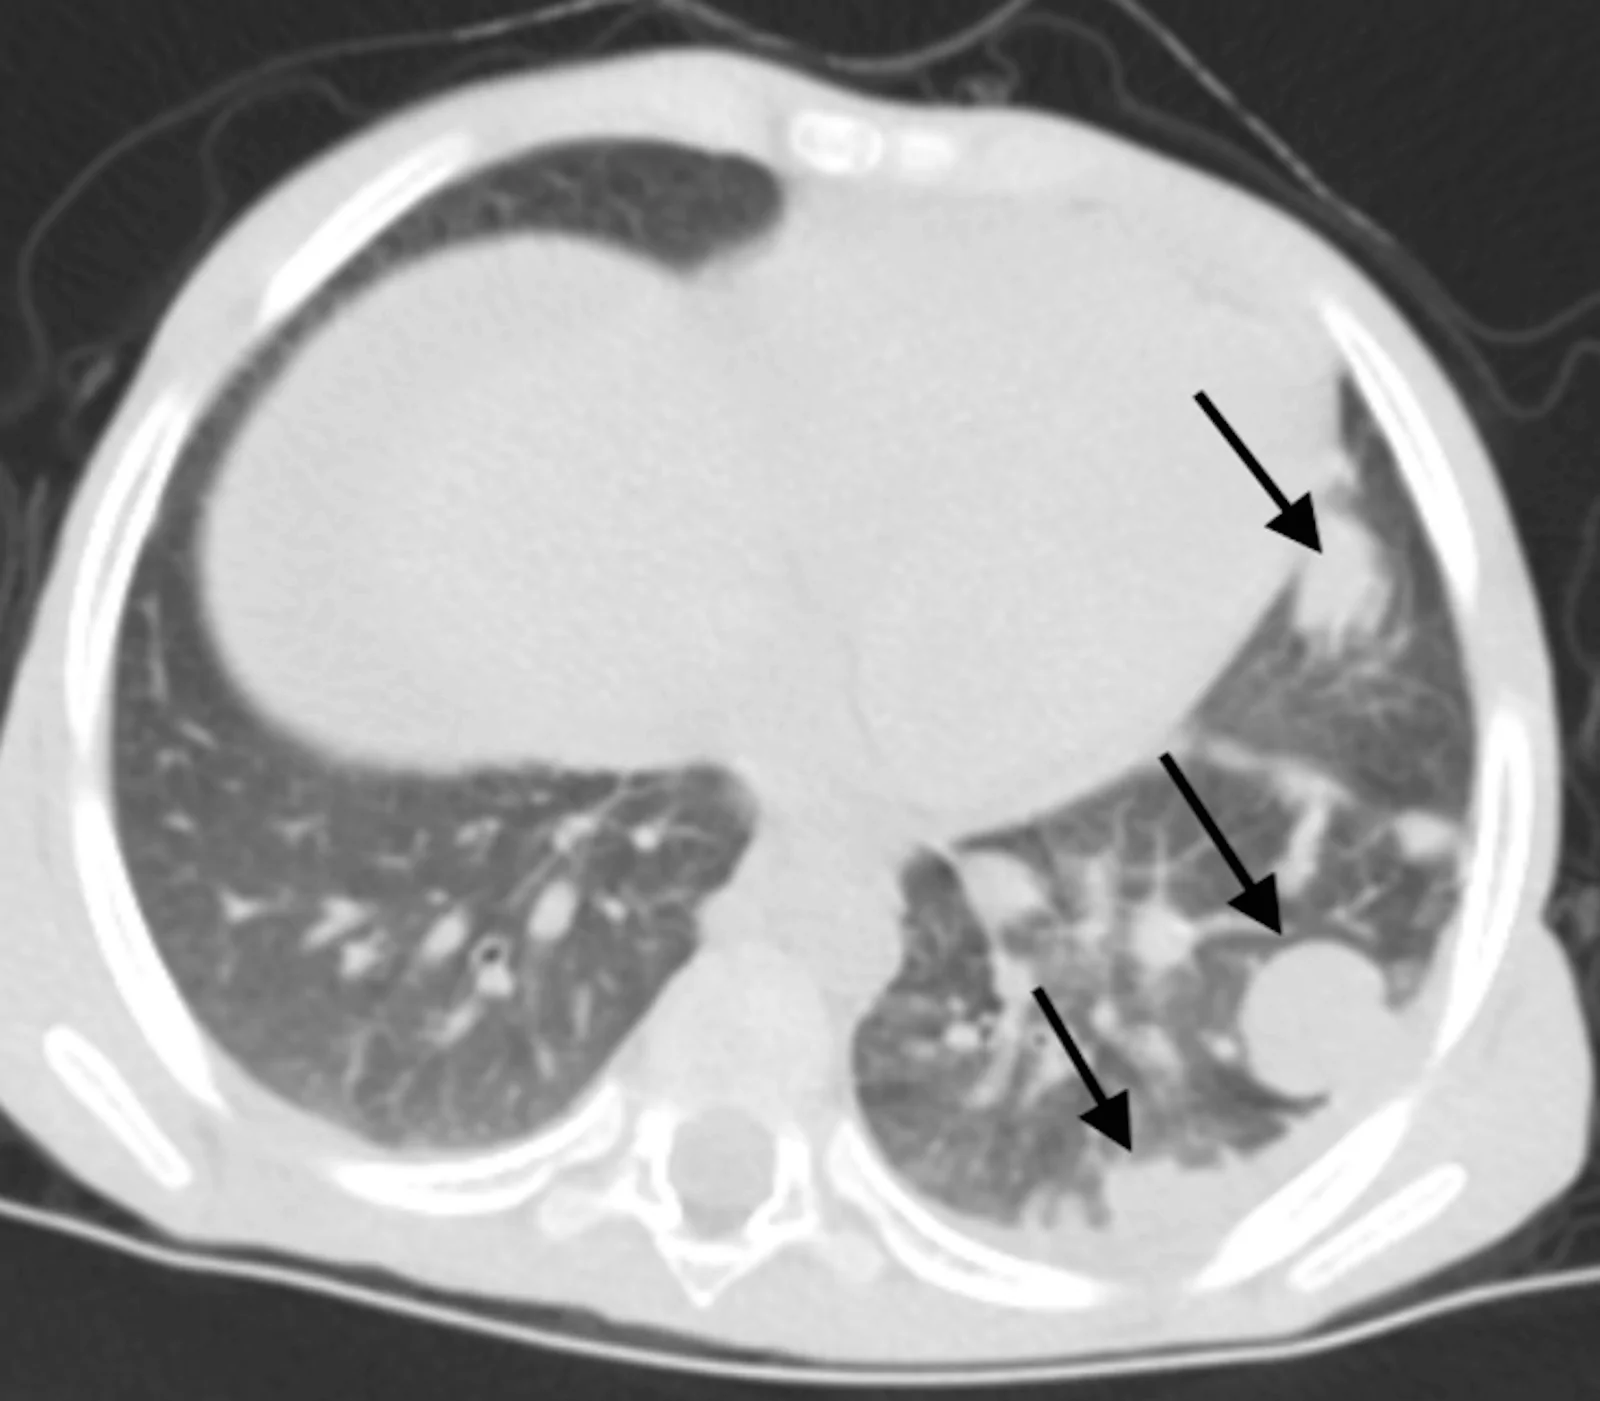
Initial chest CT, lung window. Multiple bilateral subpleural pulmonary nodules (black arrows) compatible with metastatic disease.
